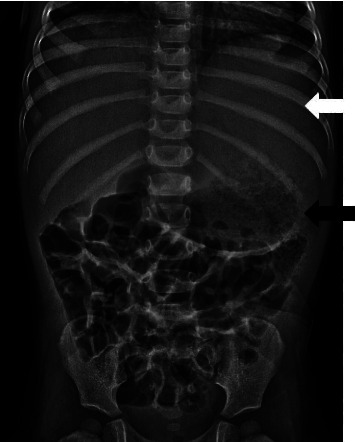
Anteroposterior supine abdominal radiograph shows abnormal soft tissue density in the left upper quadrant (white arrow) with inferior displacement of the stomach bubble (black arrow).
